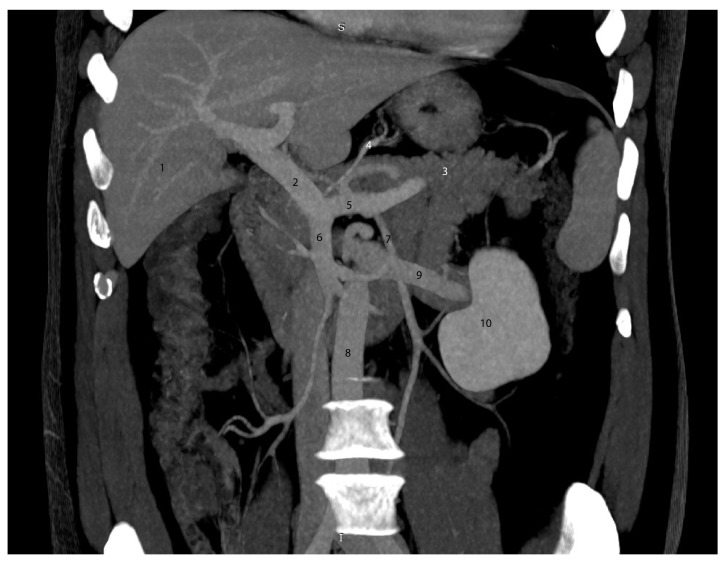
Mesenteric vein confluence—CT MPR reconstruction in the coronal plane during the venous phase, with 26 mm MIP (maximum intensity projection). 1—Liver; 2—portal vein; 3—pancreas; 4—left gastric vein vein; 5—splenic vein; 6 – superior mesenteric vein; 7 – inferior mesenteric vein; 8 – inferior vena cava; 9 – left renal vein; 10 – left kidney.
